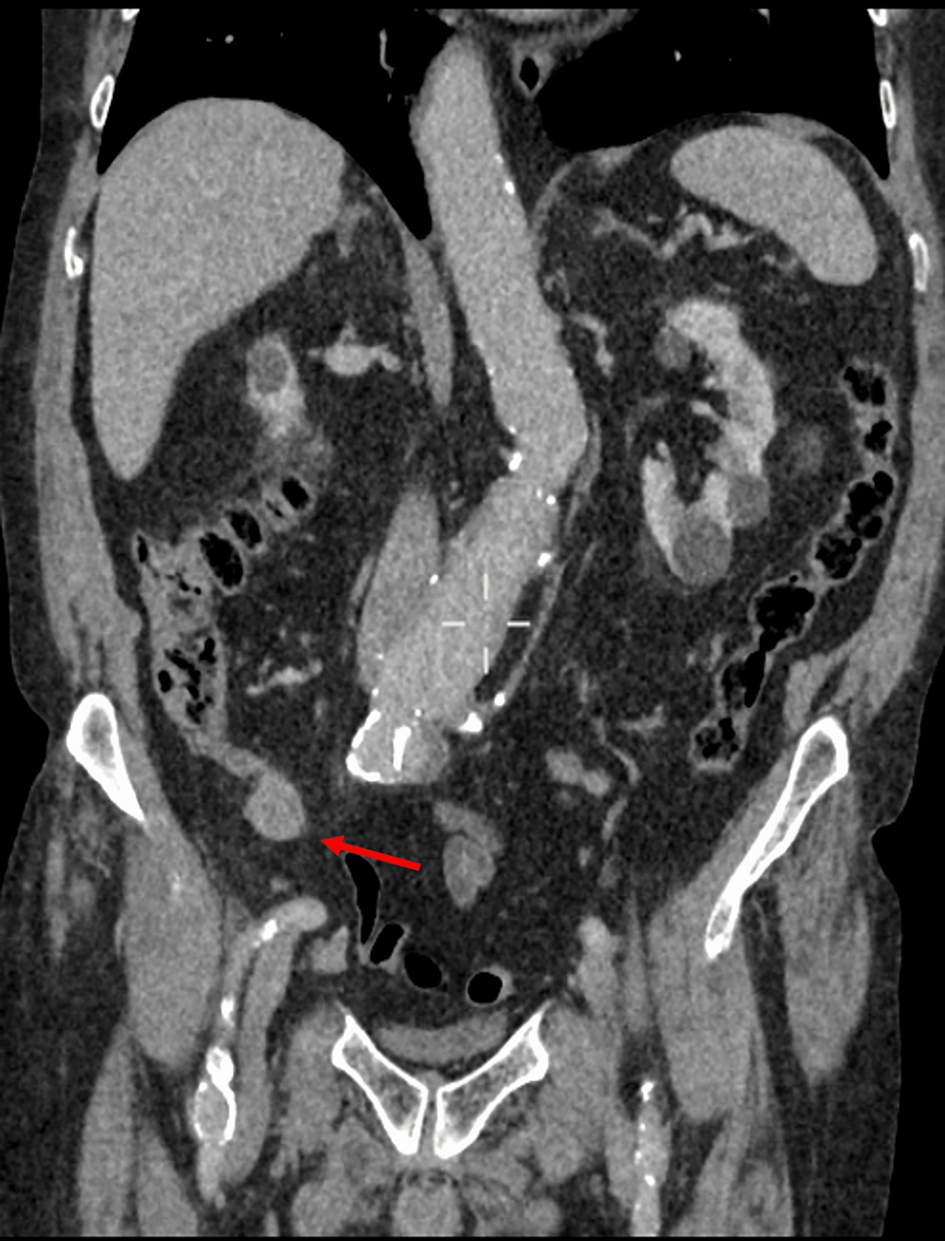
CT of the abdomen and pelvis - coronal section. The coronal cross-sectional CT image of this patient showing a fusiform appendiceal mass (red arrow) with no evidence of an inflammatory process or appendiceal obstruction.
